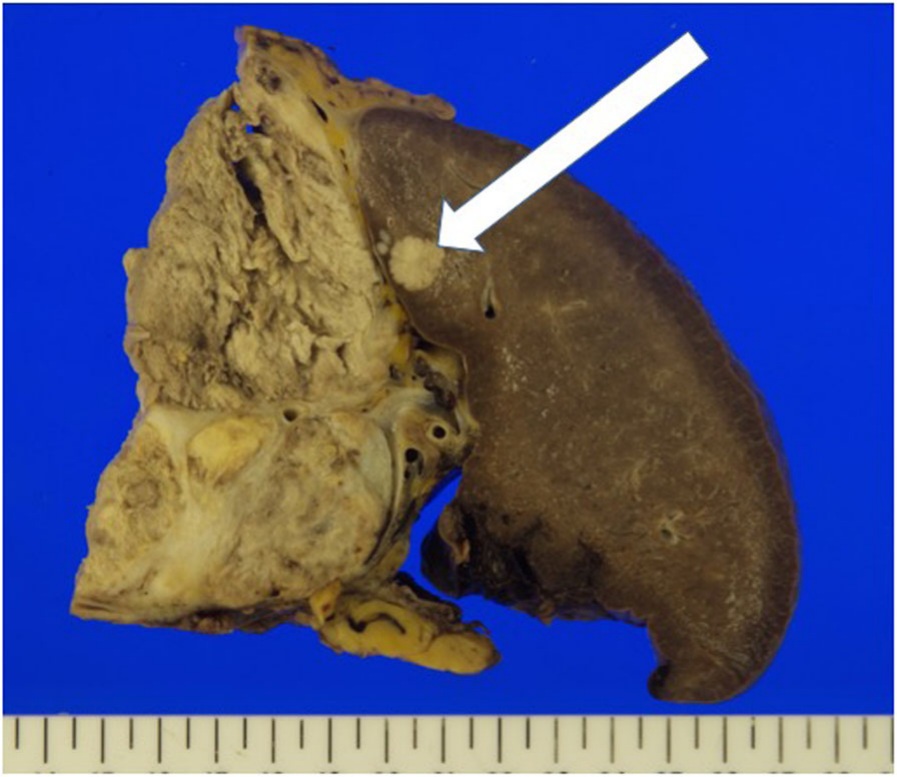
Gross pathology image of the cut surface of the spleen and tumor showing a 1.0 cm metastasis (arrow), corresponding to the lesion seen on the computed tomography scan
